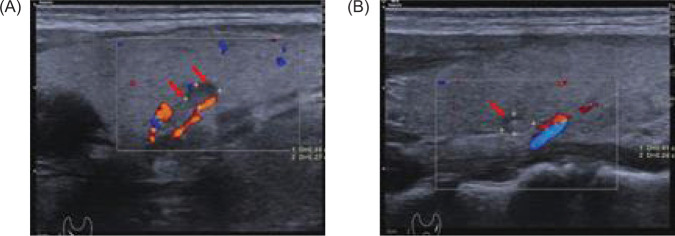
Thyroid color Doppler ultrasound shows bilateral cystic and solid thyroid nodules with clear boundaries, measuring (A) about 0.44×0.27 cm (right) (red arrow), and (B) 0.41×0.24 cm (left) (red arrow) (C-TIRADS: category 3).
